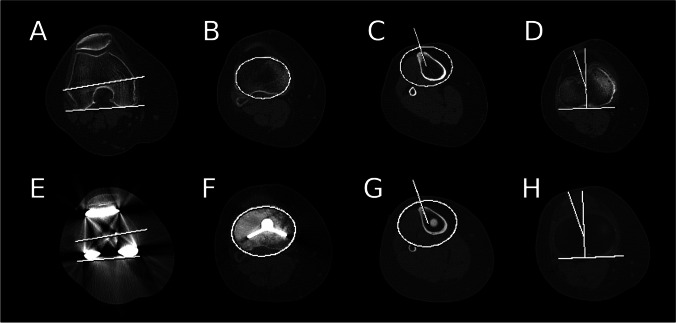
Screen capture of axial CT images including electronic calipers and goniometers demonstrating the method of measurement of preoperative femoral (A) and tibial version (B–D), and postoperative femoral (E) and tibial TKR component rotation (F–H)
